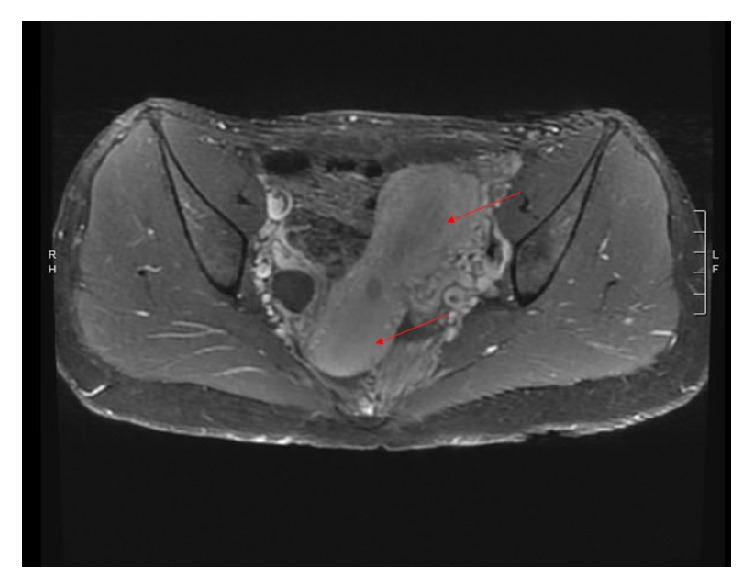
MRI demonstrating bicornuate uterus.
